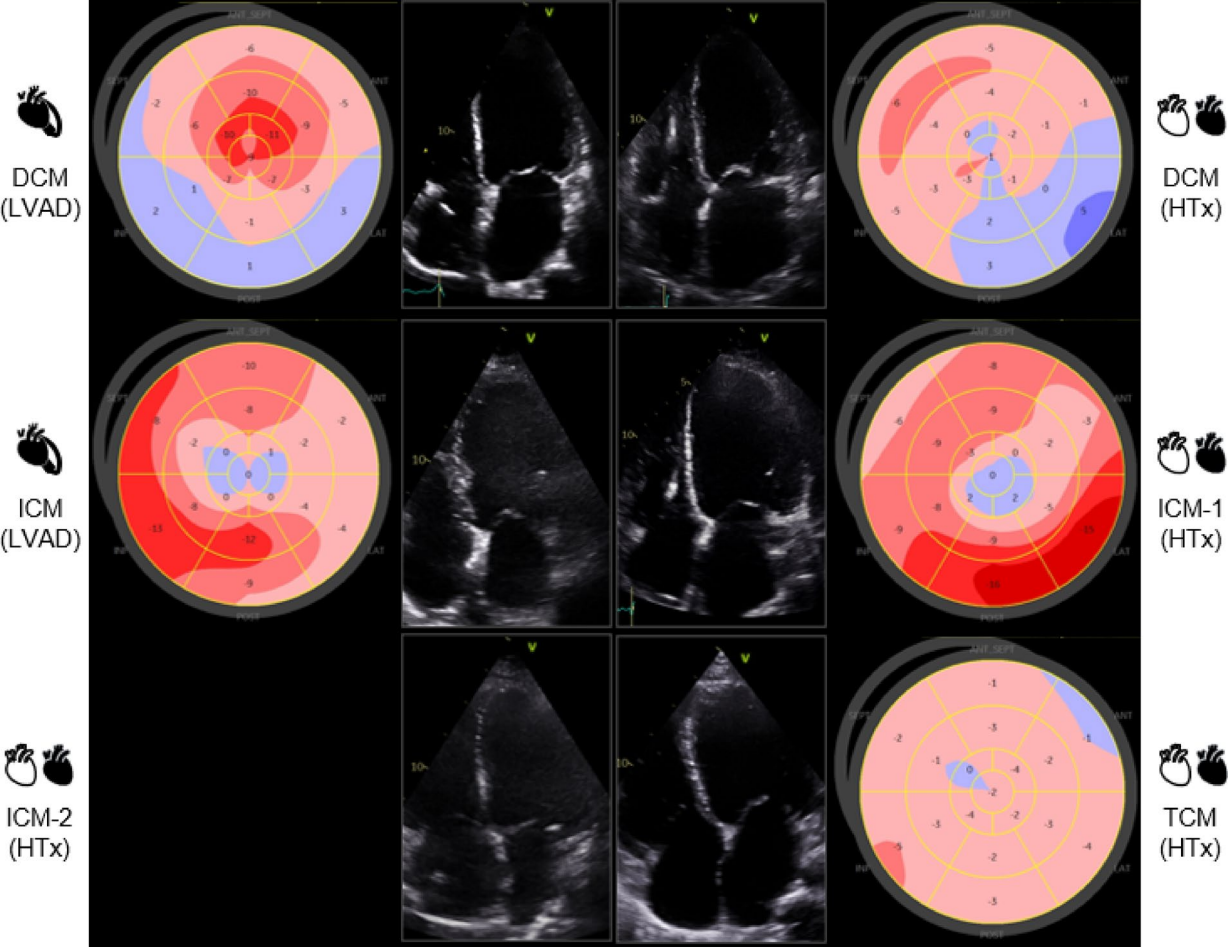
Non-invasive imaging phenotypes. The four-chamber apical view (central images) shows that the structural phenotype of the heart is similar in end-stage HF (i.e., eccentric remodelling, enlarged LV end-diastolic diameter and volume, enlarged LA), whereas the global longitudinal strain bullseye plots (left and right columns) show different functional phenotypes associated with disease aetiologies (i.e., regional differences in peak systolic deformation in ischaemic cardiomyopathy related to the coronary blood flow distribution vs. global deformation impairment in dilated and toxic cardiomyopathies). Patient ICM-2 had a poor echocardiographic window with image quality that did not permit deformation analysis. *HF* heart failure, *LV* left ventricle, *LA* left atrium, *DCM* dilated cardiomyopathy, *ICM* ischaemic cardiomyopathy, *TCM* toxic cardiomyopathy.
